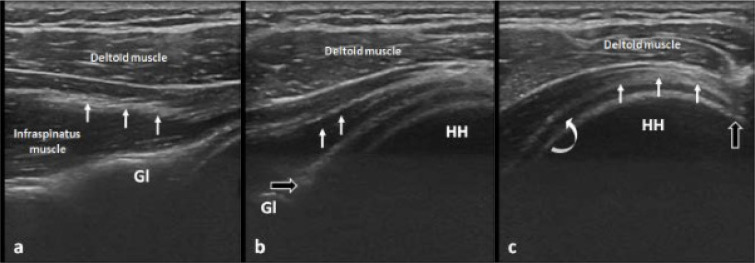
(a) Sonographic image along the long axis of the infraspinatus muscle obtained by placing the probe just inferior and parallel to the scapular spine, showing the infraspinatus central tendon (arrows) lying superficial to the glenoid (Gl). (b) Sonographic image obtained by moving the transducer lateral to “a” shows the infraspinatus myotendinous junction (white arrows) at the level of the posterior joint recess overlying the posterior labrum (black arrow), glenoid (Gl) and medial part of the humeral head (HH). (c) Sonographic image obtained by moving the transducer lateral to “b” shows the infraspinatus tendon (arrows) overlying the articular cartilage (curved arrow) of the humeral head (HH) and inserting on the greater tuberosity (black arrow).
